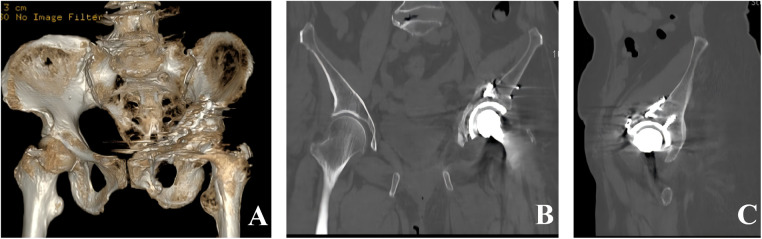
Postoperative CT images of the pelvis at 6 months postoperatively. (A) Three-dimensional reconstruction. (B) Coronal CT image. (C) Sagittal CT image.
